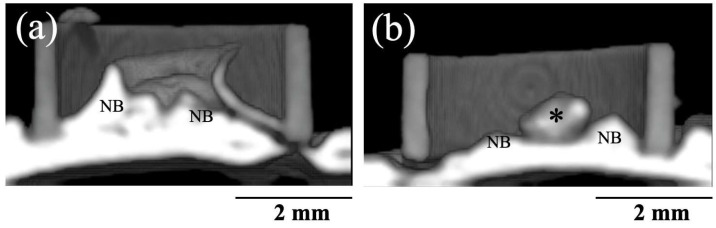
Three-dimensional CT images of new bone formations into PTFE tubes by implantation of BAp and HAp paste 8 weeks after surgery. (a) BAp paste, (b) HAp paste. NB: Newly formed bone, Asterisk: residual apatite.
